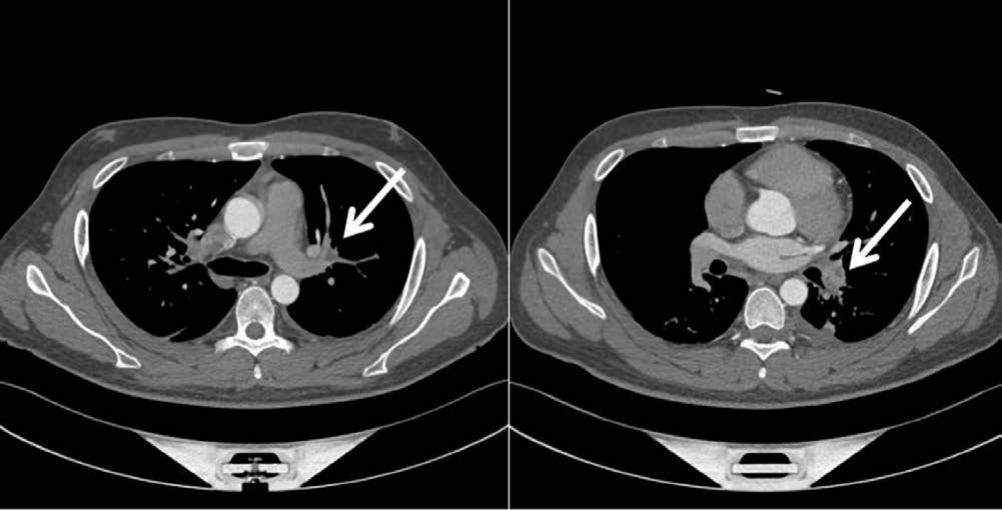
CTA of the pulmonary artery (the white arrow shows the embolism). CTA = computed tomography angiography.
